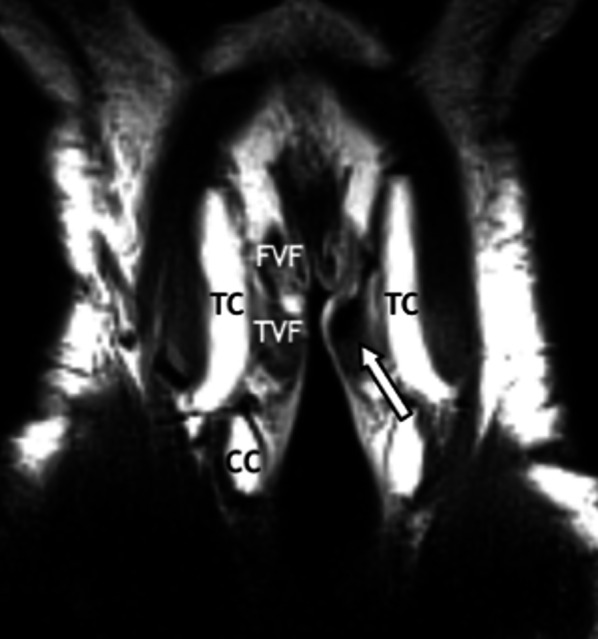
Fibrosis of the left true vocal fold (arrow) in a 60-year-old man 3 years after ChT/RT for laryngeal cancer. Coronal T2-weighted MR image acquired with surface-coil MRI of the larynx shows hypointense signal of the left true vocal fold. TC, thyroid cartilage; CC, cricoid cartilage; FVF, false vocal fold; TVF, true vocal fold
